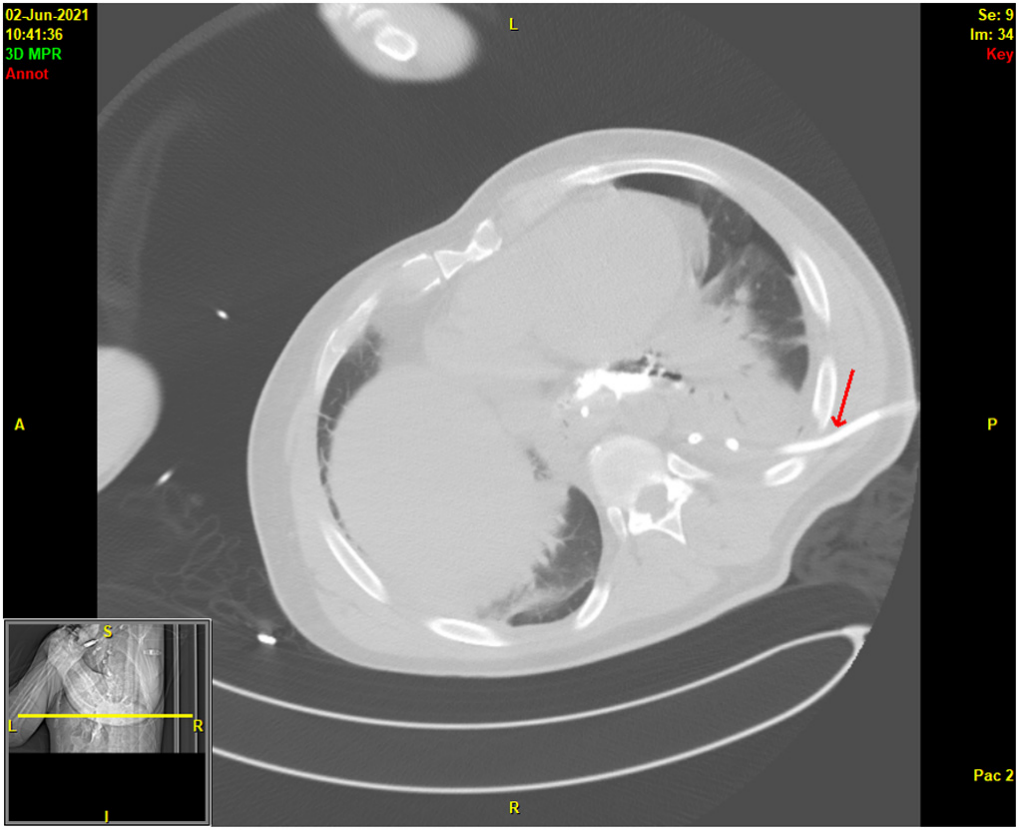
Chest CT scan showing placement of a percutaneous drain for the mediastinal air and fluid-filled collection.
